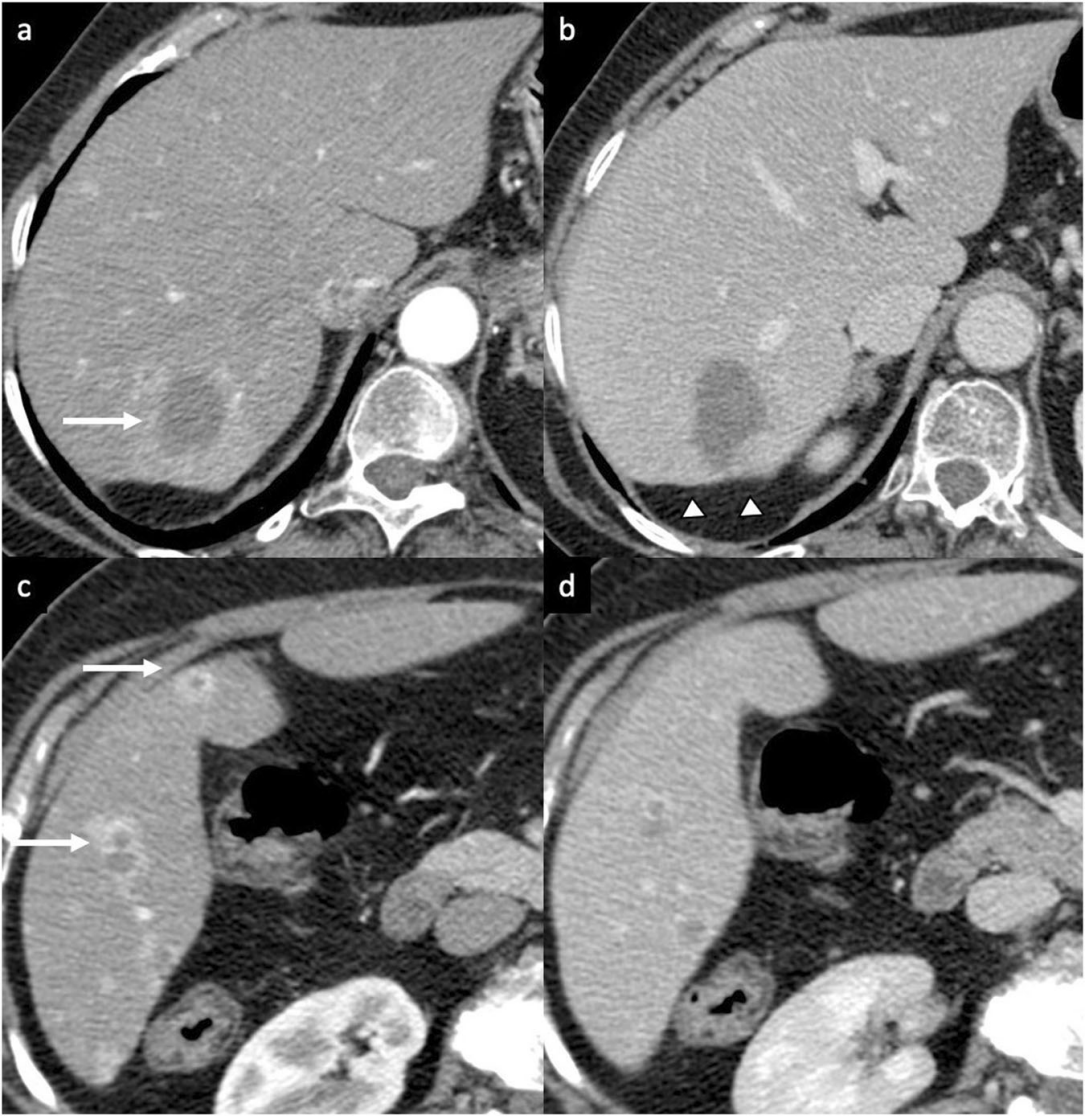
EHE in a 66-year-old woman. a, c Contrast-enhanced CT shows multiple liver lesions with nodular or irregular rim APHE (arrows) with (b, d) gradual enhancement on the portal venous phase. The lesions predominate in the peripheral regions of the liver (subcapsular). Note, the minimal retraction of the liver capsule (b, arrowheads). A biopsy of the liver confirmed the diagnosis of EHE
